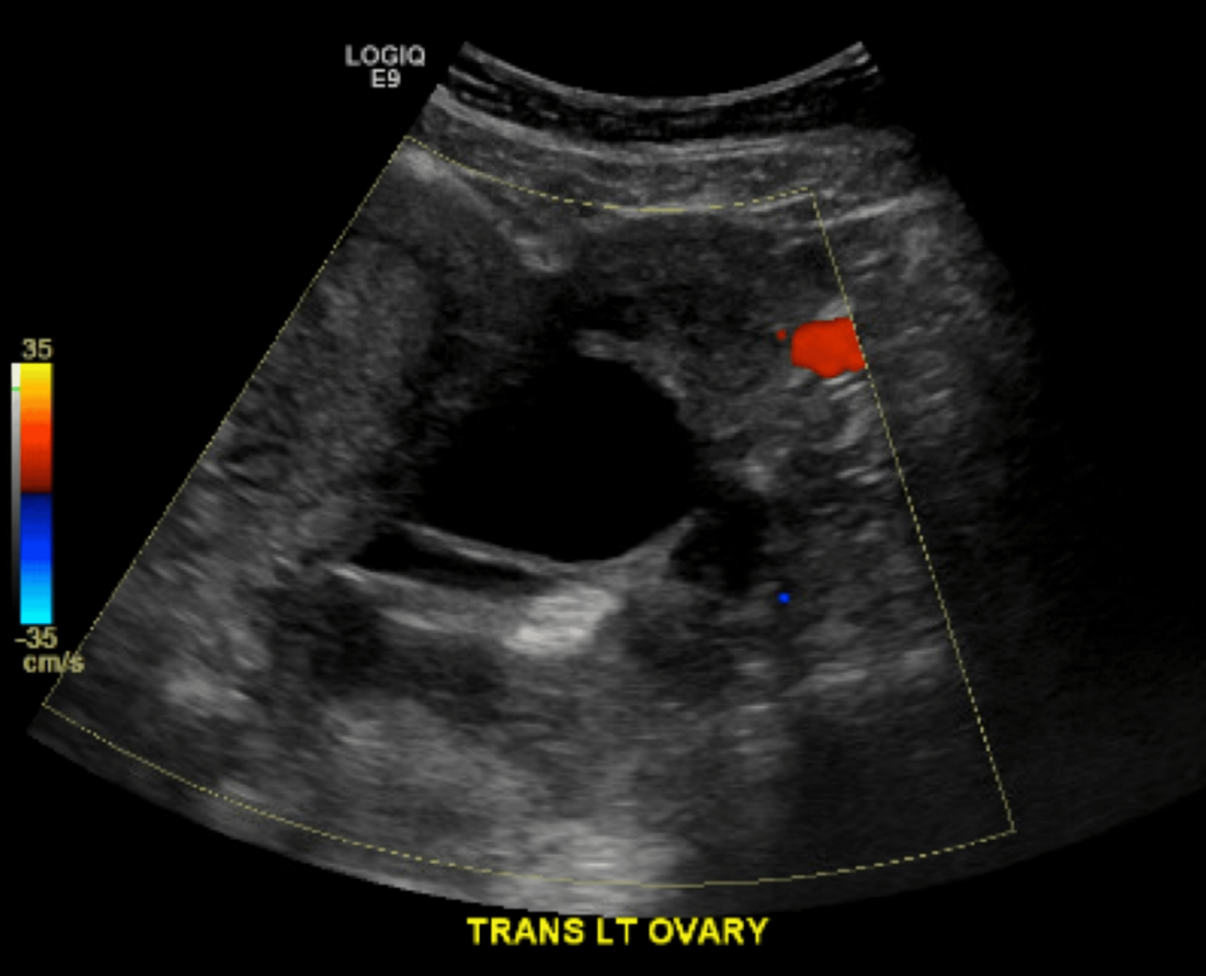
Ultrasound transvaginal view of the left ovary This is another transvaginal view of the left ovary showing the size, cystic focus, and lesion.
